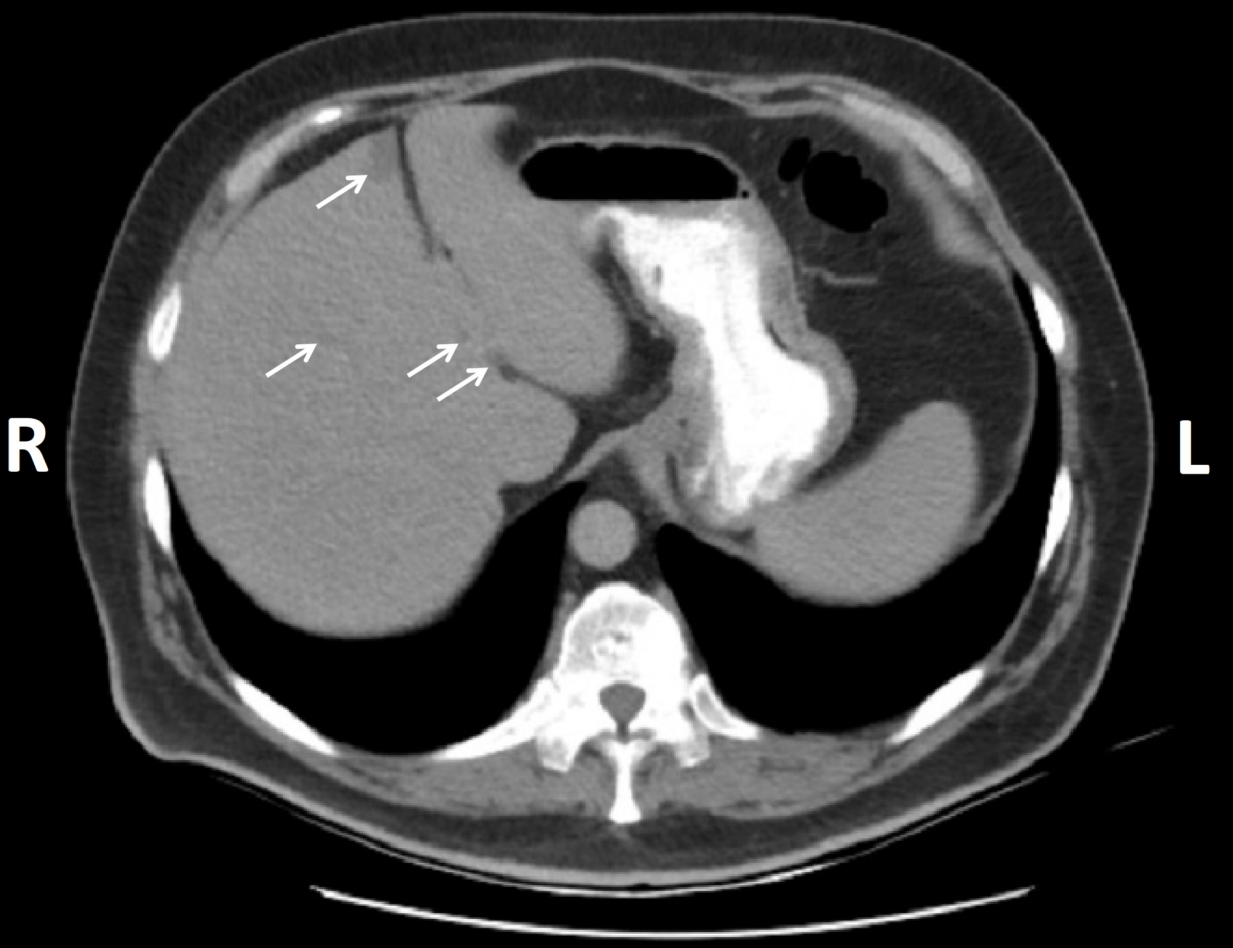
Abdominal CT scan showing hepatic focal hypodense hepatic lesions (white arrows) R: right; L: left
